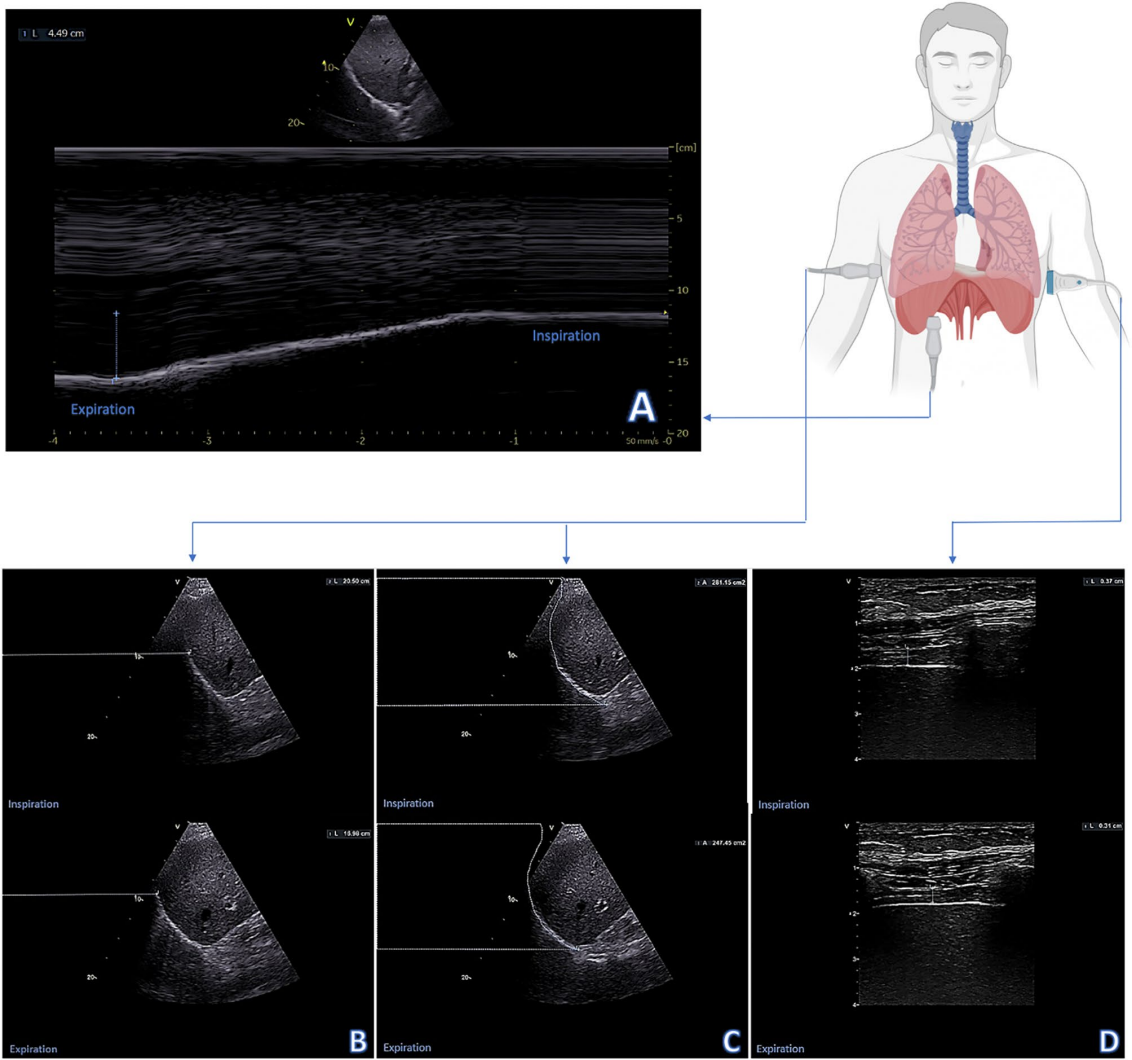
Ultrasonographic endpoint. A Excursion of the posterior part of the right hemidiaphragm performed between the midaxillary and midclavicular line. B Excursion of the hemidiaphragmatic top point in the midaxillary line. C Change in intrathoracic area of the hemidiaphragm performed in the midaxillary line. D Diaphragmatic thickness of the right hemidiaphragm
